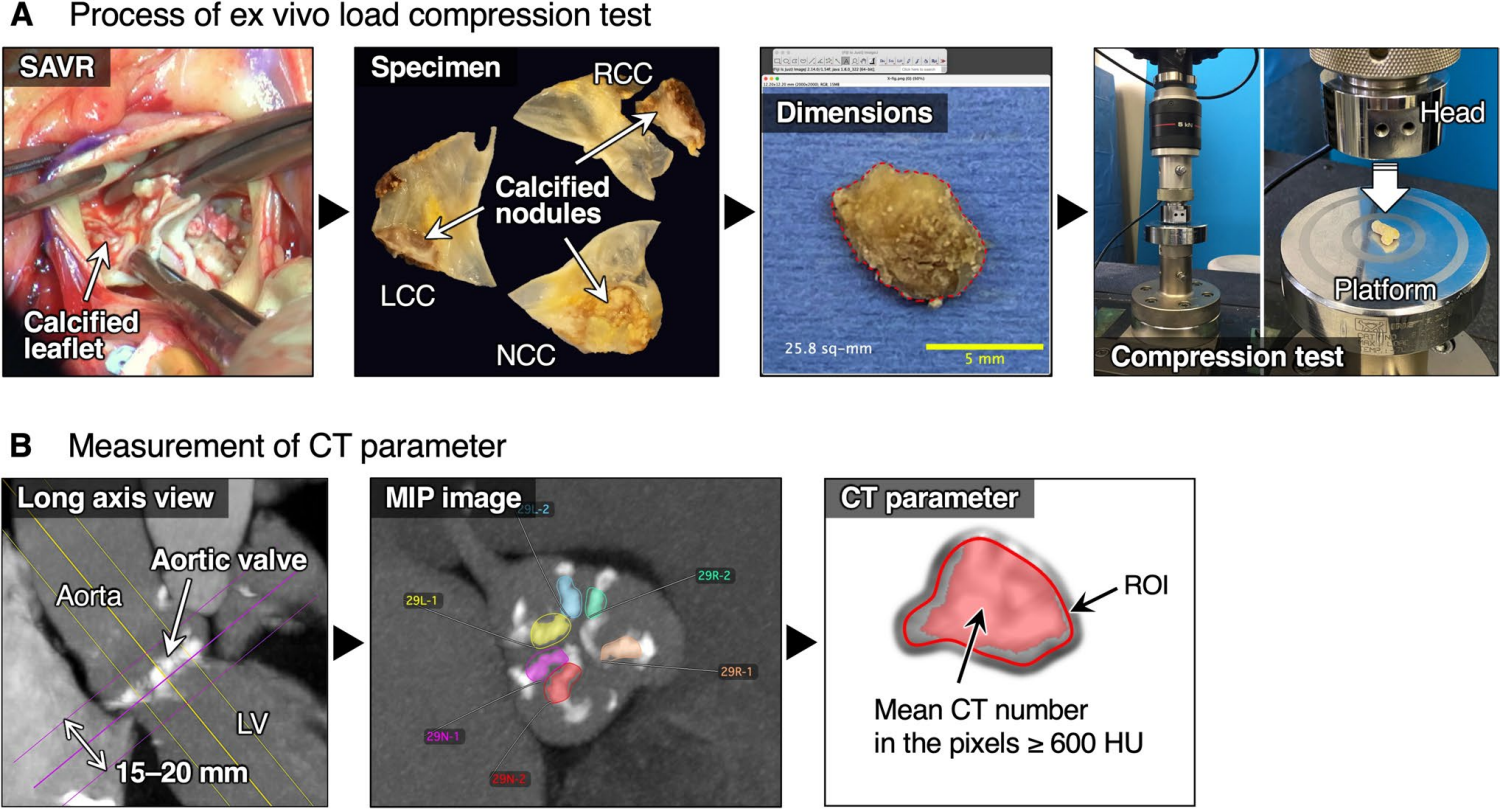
Ex vivo load compression test and CT density. A Calcified aortic valve leaflets were resected during SAVR. Intact calcified nodules without visible damage were isolated from the resected valves. Nodule height was measured using calipers, and projected area was determined from frontal images using image analysis software. Each calcified nodule was subjected to compression testing using a load testing machine. B A 15–20-mm-thick slab parallel to the virtual basal ring was used to encompass all valvular calcifications. ROIs were defined on the generated MIP image to enclose the corresponding calcified nodules. The mean CT number was calculated in high-density pixels with CT values ≥ 600 HU within each ROI
